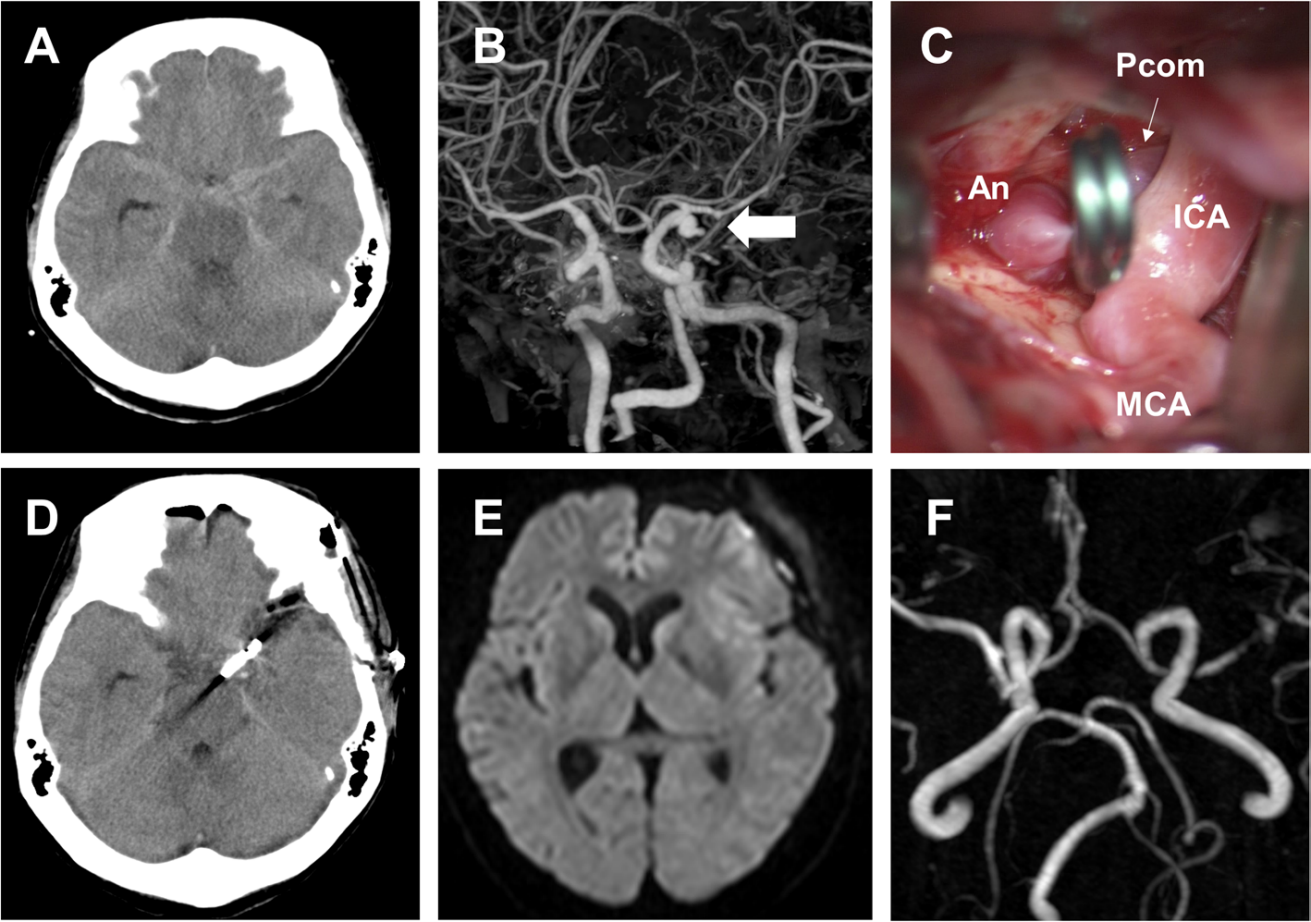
Imaging findings. a Computed tomography (CT) scan of the brain revealing subarachnoid hemorrhage. b CT angiographic view showing an aneurysm of the posterior communicating artery branching from the left internal carotid artery (*arrow*). c Intraoperative view showing a ruptured aneurysm* with bleb. d Postoperative axial CT imaging showing Sugita clip and no additional bleeding. e and f A diffusion-weighed magnetic resonance (MR) imaging and MR angiographical scan showing the absence of cerebral infarction or cerebral vasospasm. *An, aneurysm; ICA, internal carotid artery; MCA, middle cerebral artery; Pcom, posterior communicating artery
